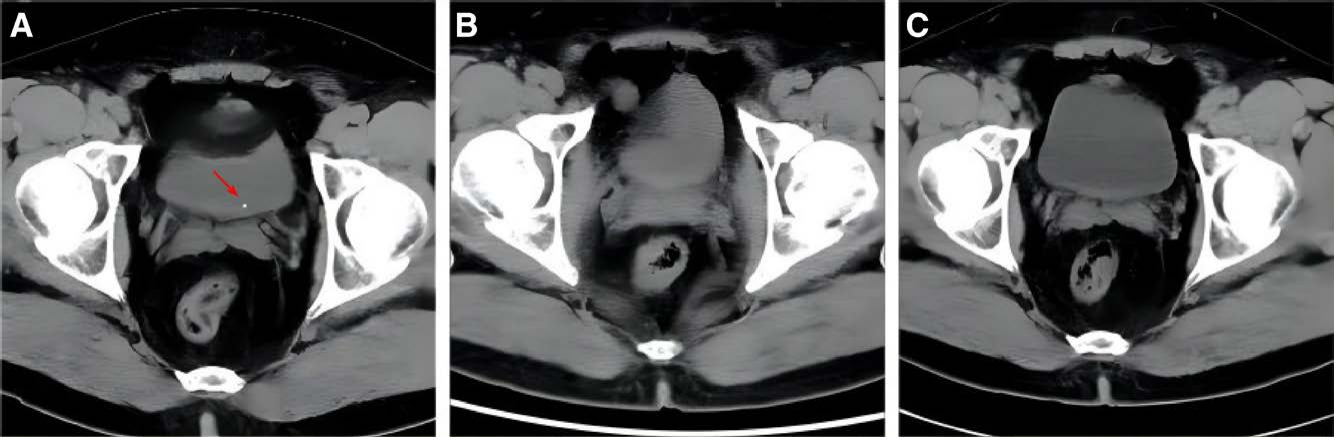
CT of urinary tract imaging on March 27, 2022: a small calculus at the distal end of the left ureter (within the bladder wall), approximately 2 mm in size, with mild hydronephrosis in the ureter and renal pelvis. (A) CT of urinary tract imaging on May 19, 2022: no urinary tract stones found. (B) CT of urinary tract imaging on April 14, 2023: no urinary tract stones found. (C) Detailed CT images can be found in the supplementary materials. CT = computed tomography.
